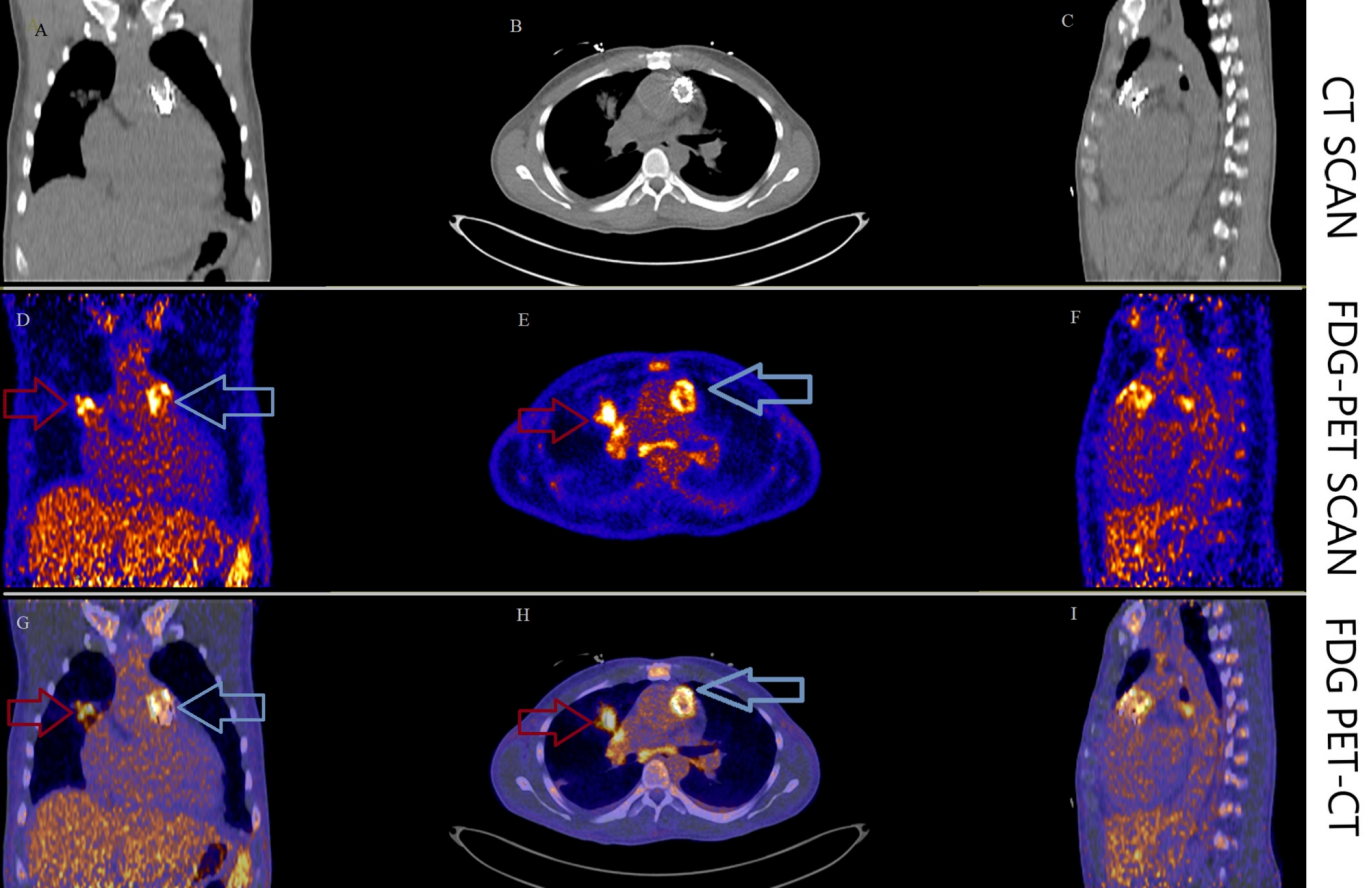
FDG PET/CT scan of the chest showing increased 18F uptake in the region of the Melody valve indicating PVE (blue arrows in panels D, E, G, H) and pulmonary septic embolus (red arrows in panels D, E, G, H). Coronal, transverse, and sagittal sections of the chest using CT scan (panels A, B, C), FDG-PET scan (panels D, E, F) and FDG PET-CT fusion (panels G, H, I). ^F^18 FDG uptake is noted in the region of the pulmonic Melody valve (blue arrows) consistent with the clinical diagnosis of infective endocarditis. Multiple areas of ^F^18 FDG uptake are noted in both lungs, one in the right hilar region (red arrows) and several in the subpleural region consistent with pulmonary septic emboli. FDG PET/CT: flourodeoxyglucose positron emission tomography-computerized tomography scan; PVE: prosthetic valve endocarditis
